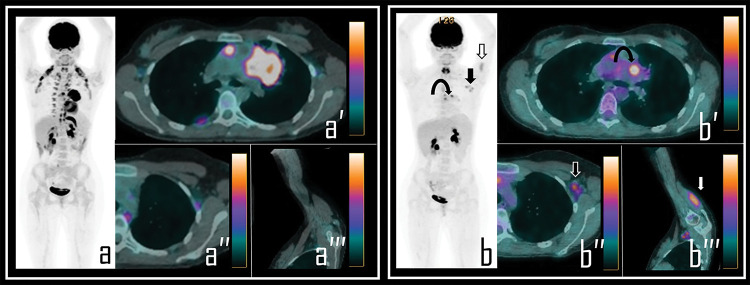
A 36-year-old male patient examined by 2-[^18^F]FDG PET/CT during the staging of *Hodgkin’s lymphoma*. Baseline PET/CT (a) showed a mediastinal *bulky* lymphoma, more evident in axial PET/CT view (a′). No abnormal foci of uptake were observed in left axilla neither in ipsilateral deltoid muscle, as showed in axial PET/CT detail (a′′) and oblique PET/CT view of the left arm (a′′′). Note: After 2 cycles of chemotherapy, the patient underwent *interim* 2-[^18^F]FDG PET/CT, showing incomplete response to therapy in the mediastinal mass with residual lymphoid active tissue, as evident in PET *Maximum Intensity Projection* (b, *curved arrow*). PET also showed focal uptake in the left deltoid (b, *white arrow*) and in three ipsilateral axillary lymph nodes (b, *black arrow*). Residual lymphoma is evident in axial PET/CT view (b′, *curved arrow*) while axial PET/CT detail shows a 2-[^18^F]FDG-avid lymph node in left axilla (b″, *black arrow*), with SUVmax 2.5; oblique PET/CT view of the left arm displays diffuse uptake in the deltoid muscle (b′′′, *white arrow*), with SUVmax 2.8. The Patient underwent first dose of COVID-19 vaccine 9 days before the second PET/CT scan.
